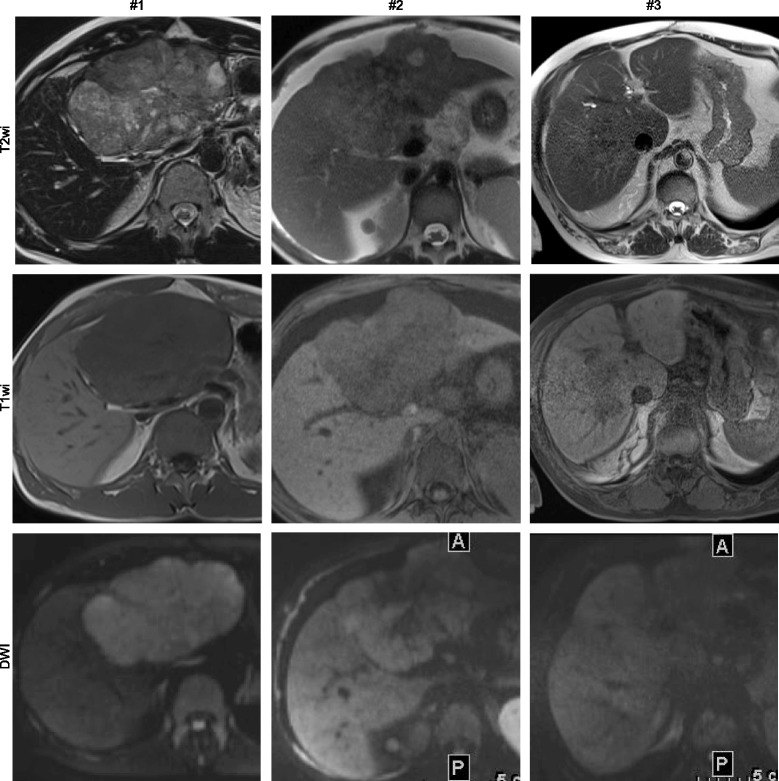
Image panel of patients #1, #2, and #3 displaying representative sections through individual fibrolamellar carcinoma in non-contrast enhanced techniques including T2 weighted imaging (T2wi), T1 weighted imaging (T1wi), and diffusion weighted imaging using high b-values (DWI)
